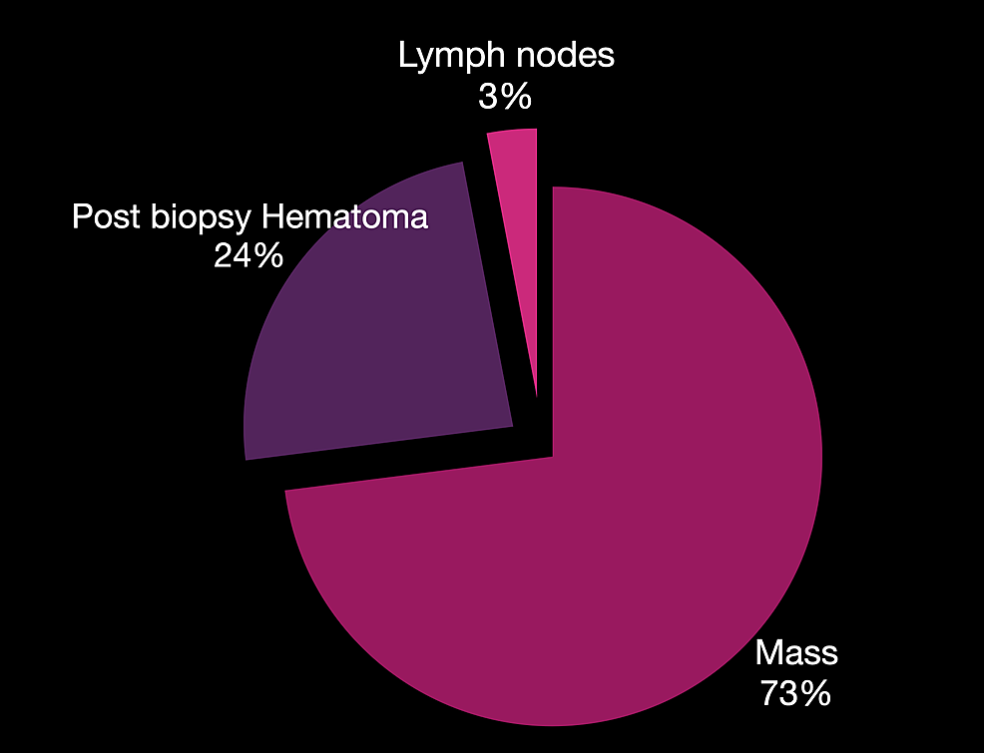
Placement sites for RFID included in our study. Mass: RFID placed into or adjacent to the ultrasound mass abnormality. Hematoma: RFID placed within a post-stereotactic biopsy hematoma. Lymph node: RFID used for localising the target lymph node. RFID: radio-frequency identifier device
